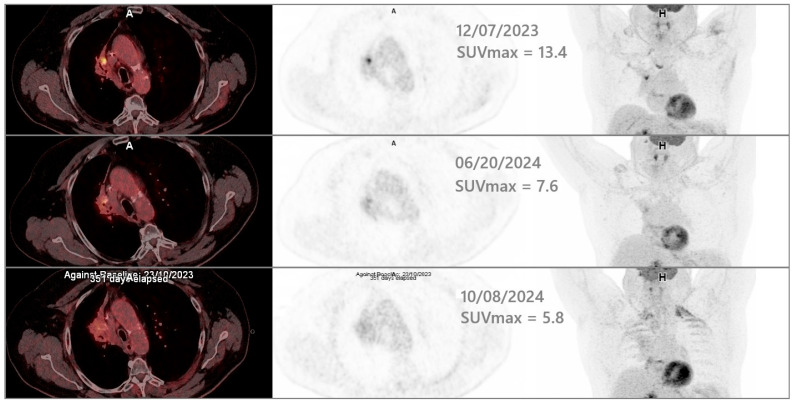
The axial and frontal MIP views from the successive time-points PET/CT. The first line shows the baseline PET/CT acquired before SBRT. The second line shows follow-up PET/CT conducted 3 months after the end of SBRT administration. The third line shows follow-up PET/CT conducted 7 months after the end of SBRT administration.
